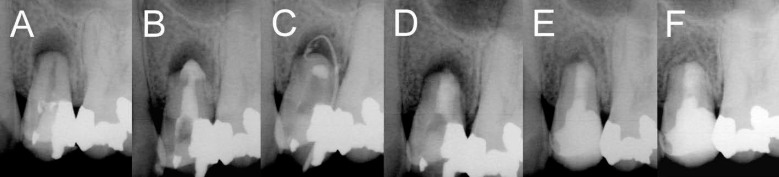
A)* Extensive IERR associated with a periapical lesion; *B)* Placing inter-appointment calcium hydroxide (CH) dressing after drainage; *C)* Formation of a sinus tract after one week; *D)* Radiographic confirmation of accurate tooth replantation after extraoral retrograde root canal filling with CEM cement; *E-F)* Radiographic healing and formation of new bone after six months *(E)* and 1 year *(F)* follow-ups
